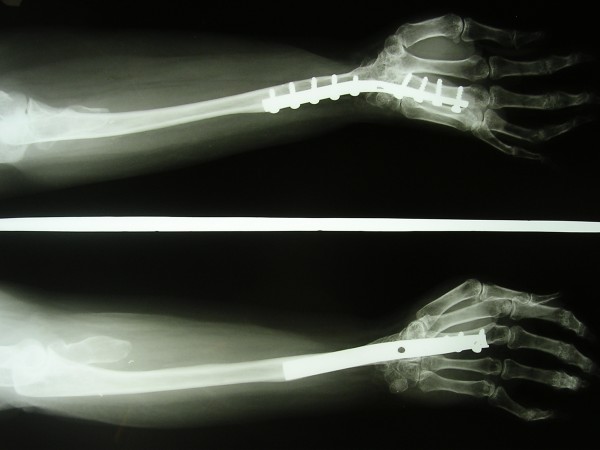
Radiograph-two years postoperative.
